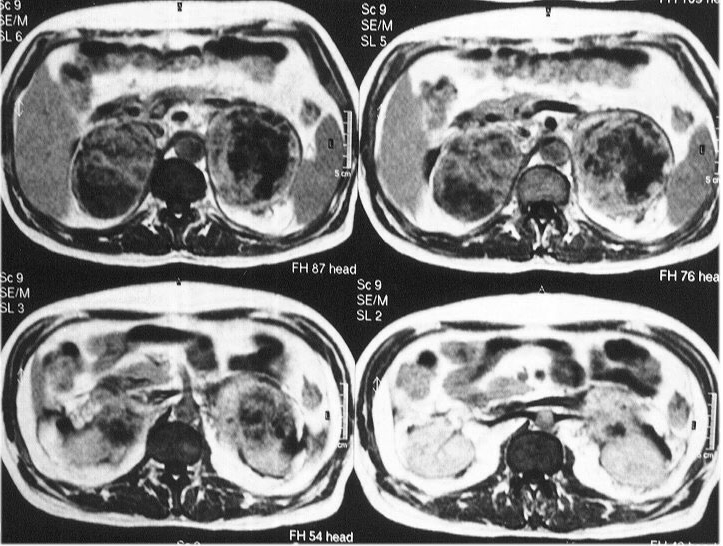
T_1 _weighted MR image showing enhancement after i.v. administration of contrast agent.
